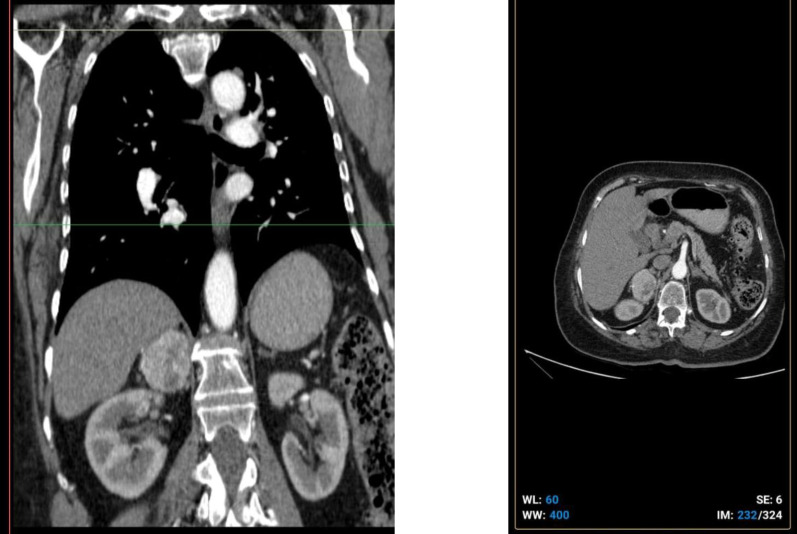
a and b: Computed tomography image showing a solid mass measuring 50 x 36 mm in the right adrenal gland.
